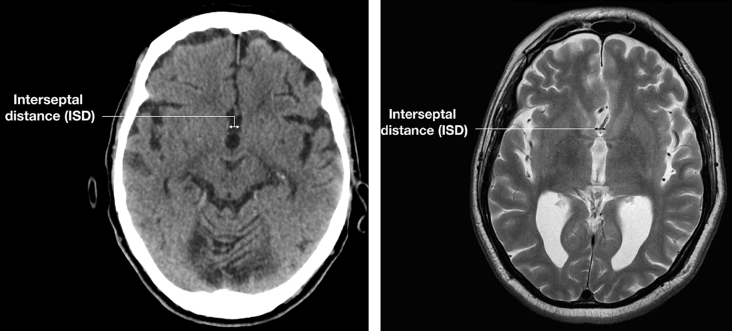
Axial CT and MRI (T2-weighted) brain images of an adult with established AD showing abnormally widened ISD.
